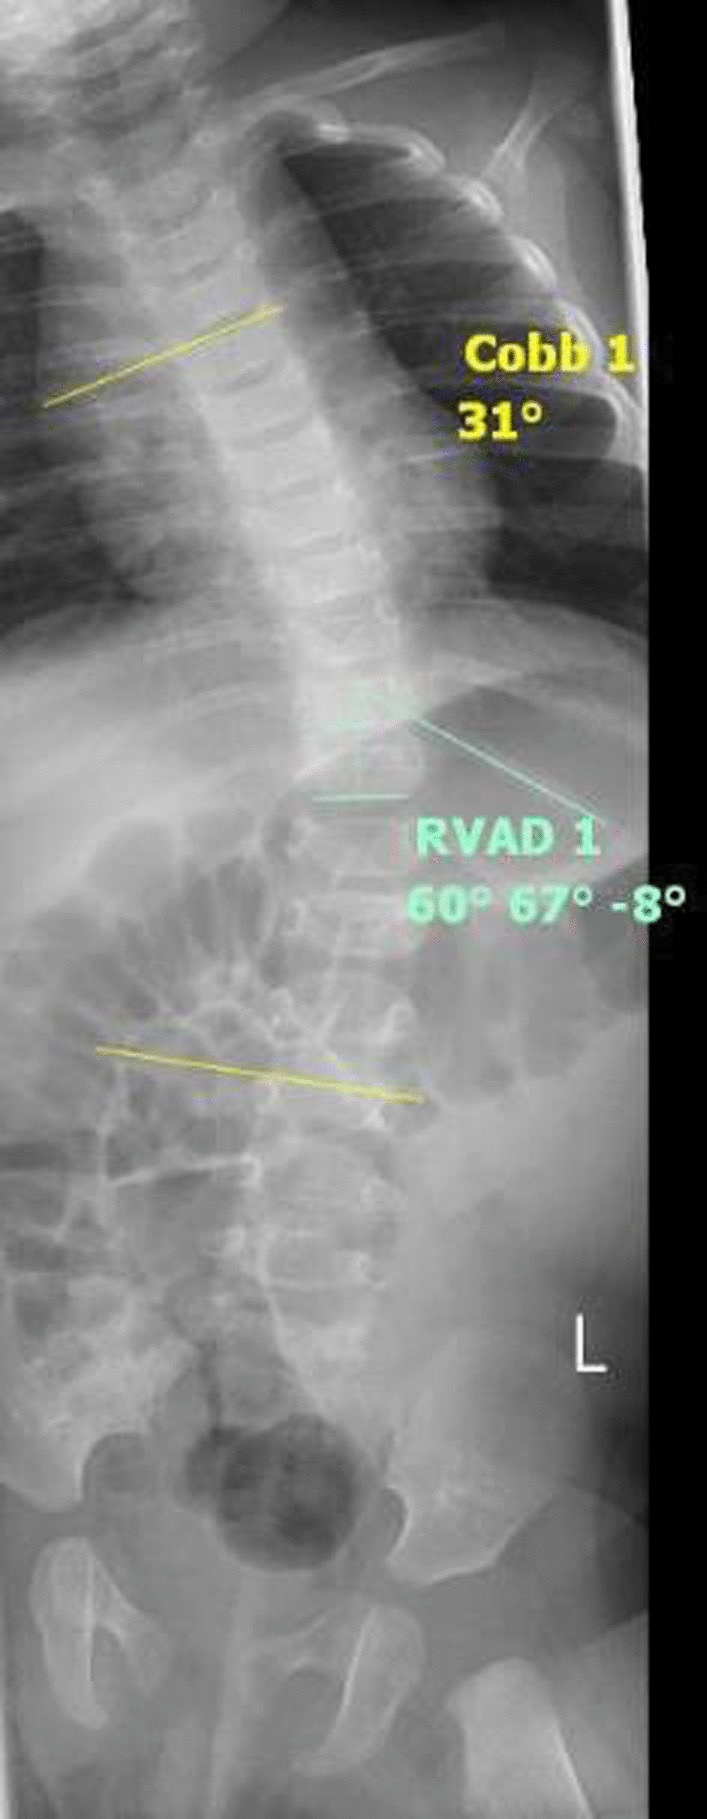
One month out-of-brace x-ray (supine anteroposterior view)
